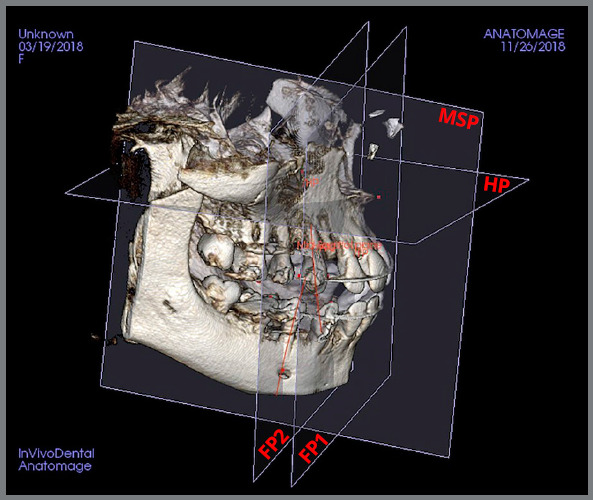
CBCT reference planes: Median Sagittal Plane (MSP), Horizontal Plane (HP), Maxillary (FP1) and Mandibular (FP2) Frontal Planes.
